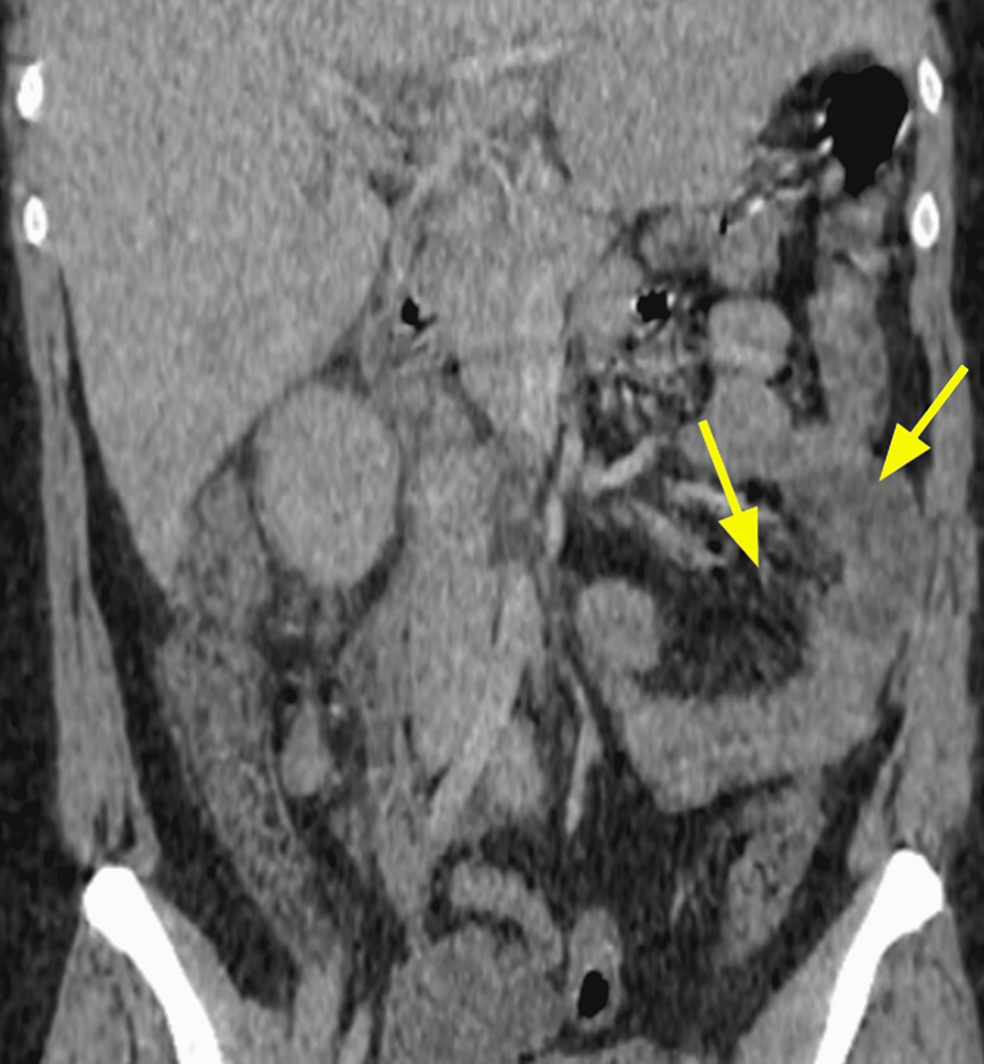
Contrast-enhanced CT image for Case 5 (coronal plane) showing thickening of left large bowel wall and infiltration of the pericolic fat (arrows)
